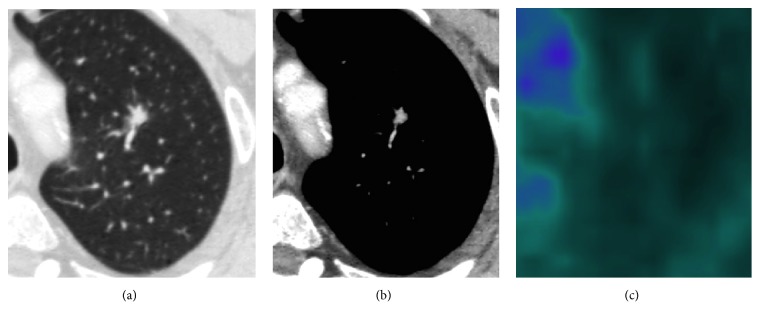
CT (a, b) and 18-FDG PET-CT (c) images of a 18-FDG PET false negative lung adenocarcinoma with lepidic predominant component; the solid nodule presented spiculated margins and notch sign (a), intense CE (88 HU) (b) despite low SUVmax (1.20) at 18-FDG PET examination (c).
